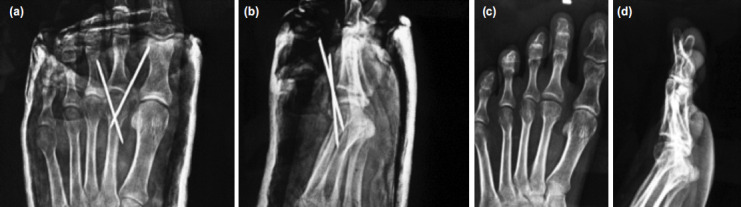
Post-operative radiographs; (a) anteroposterior, (b) lateral. Radiograph at final follow-up (c) anteroposterior and (d) lateral views.
